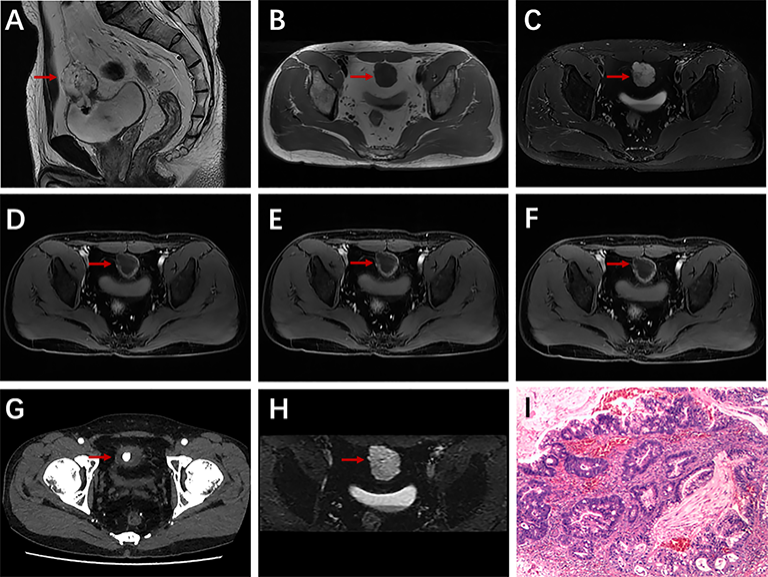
A 43-year-old man with urachal carcinoma presented with painless gross hematuria. Sagittal T2WI (A) showed a solid mass in the urachal region; very low signal intensity on T1WI (B) and very high signal intensity on T2WI (C); axial dynamic contrast-enhanced LAVA-Flex images showed apparent ring enhancement (D–F); nodular calcification on axial CT scan (G); restricted diffusion on DWI (H); pathologically confirmed urachal adenocarcinoma (intestinal type) (I).
